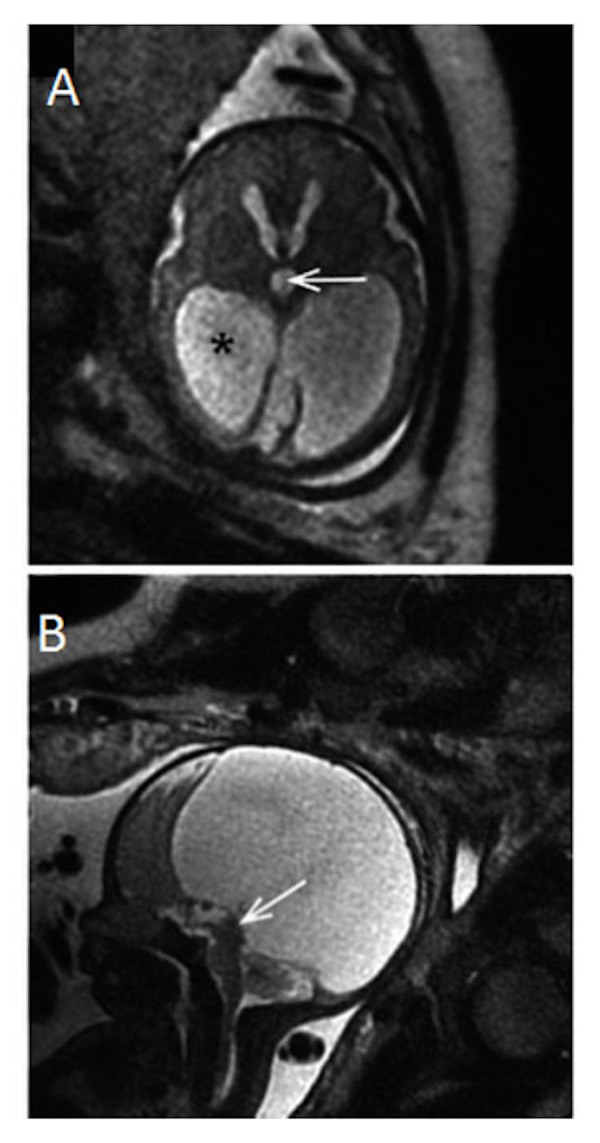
Aqueductal stenosis Panel A is a T2-weighted sagittal image in a 30-week gestational age fetus demonstrating absence of the septum pellucidum, enlargement of the third ventricle (arrow), and ventriculomegaly(*). Panel B is a T2-weighted sagittal image demonstrating lack of a fluid-filled aqueduct of Sylvius (arrow). These findings are consistent with aqueductal stenosis. Image reprinted without changes from Hosseinzadeh, et al. under the creative commons license.
